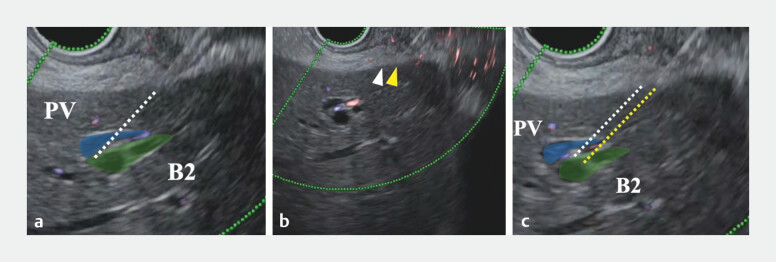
Scope withdrawal technique to prevent transesophageal puncture during intrahepatic bile duct segment B2 puncture.
